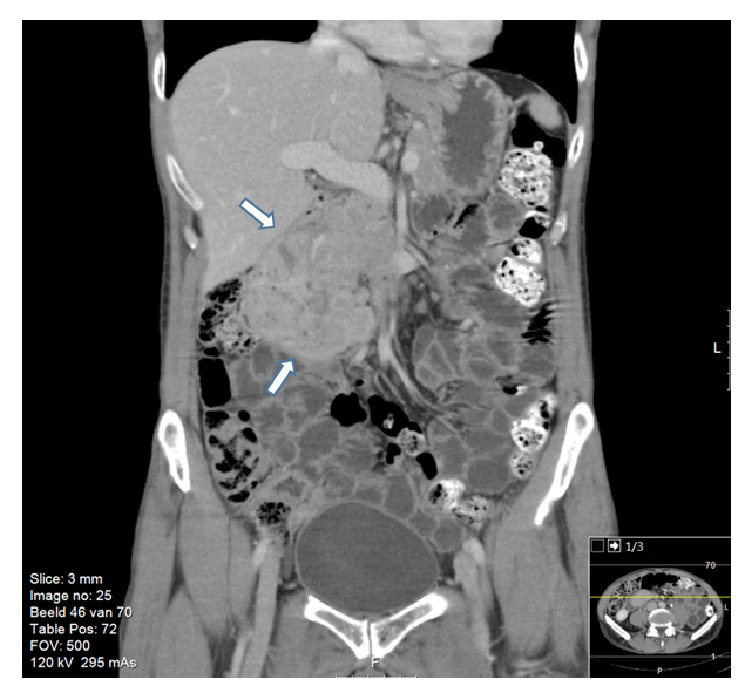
Coronal CT view showing a large mass near the proximal duodenum. Oral and IV contrast were given.
